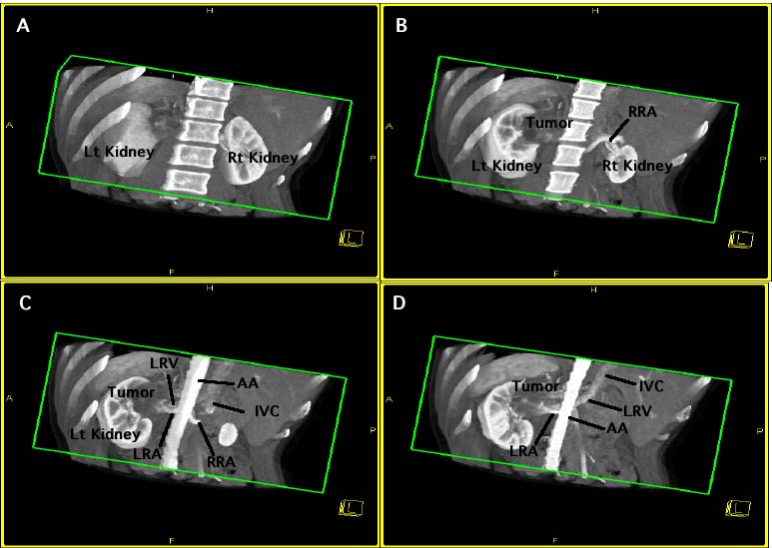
A left renal tumor adjacent to the renal artery and vein. Oblique view from the dorsal to ventral direction in the arterial phase. The CT scans are arranged from dorsal (A) to ventral (D). A: The tumor can be seen in the upper pole to middle part of the kidney. B: The tumor is more clearly visualized in the ventral images. The right renal artery (RRA) is also seen. C: The left renal artery (LRA) arises vertically from the abdominal aorta (AA), runs dorsal to the left renal vein (LRV), and drains into the inferior vena cava (IVC), indicating that the LRA and LRV cross each other antero-ventral to the tumor. D: The LRA, LRV, and IVC are seen more clearly in the ventral images.
